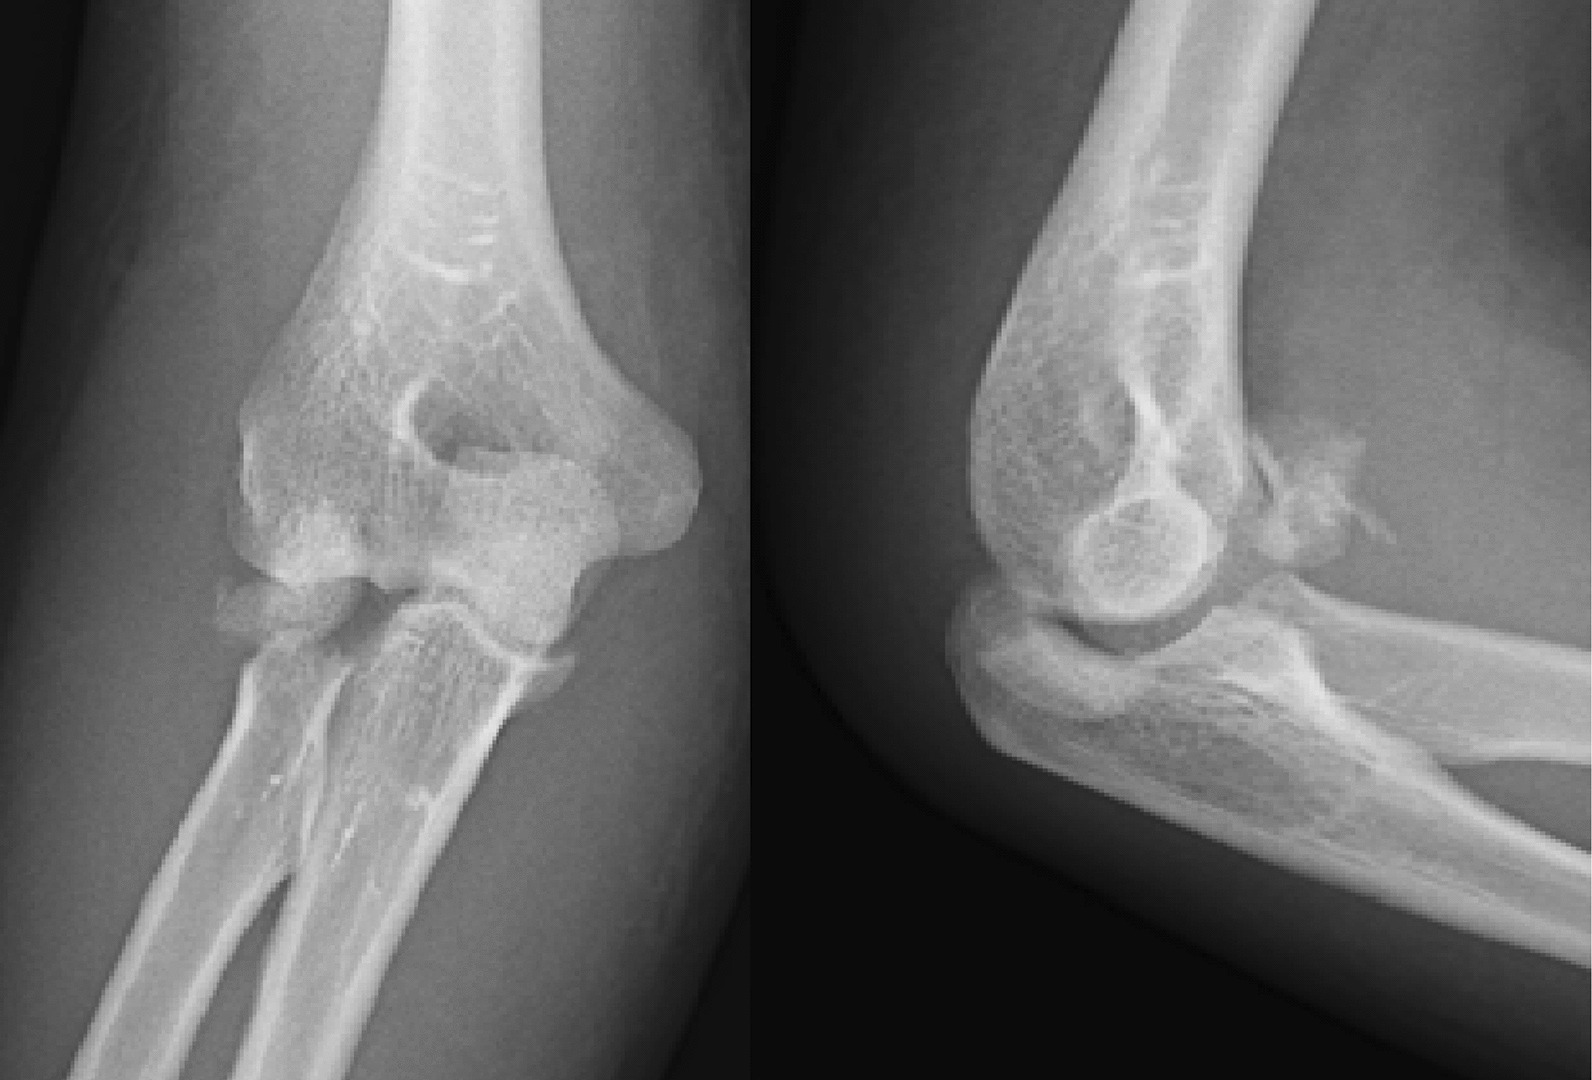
Post-reduction anteroposterior and lateral view X-ray of the right elbow
